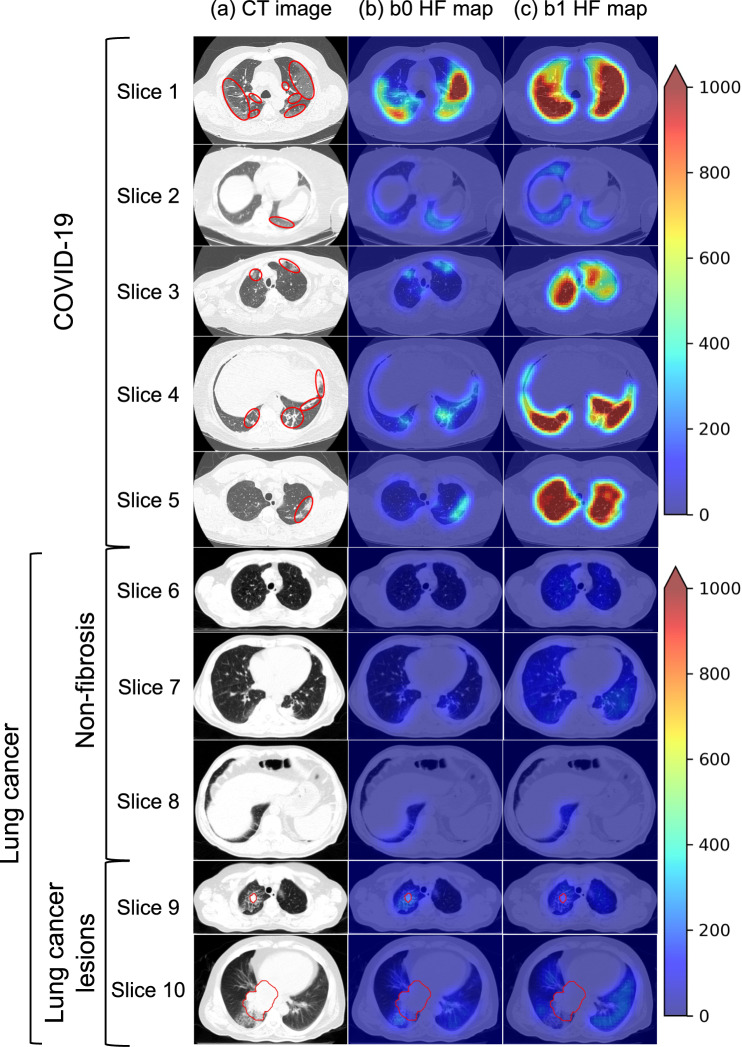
Examples of the HF maps. a, b and c show the CT image, b0 HF map, and b1 HF map, respectively. The area annotated with the red line in the CT images indicates fibrotic lesions. The colorbar presents the absolute HF map value. Here, the HF map value is adjusted from 0 to 1000 to compare the b0 HF and b1 HF maps with the same scale. The HF maps show that b0 is superior to b1 in quantifying fibrotic lesions in the CT images. Red line indicates lung cancer lesions defined by radiologists. Abbreviations: CT, computed tomography; HF, homology-based feature
